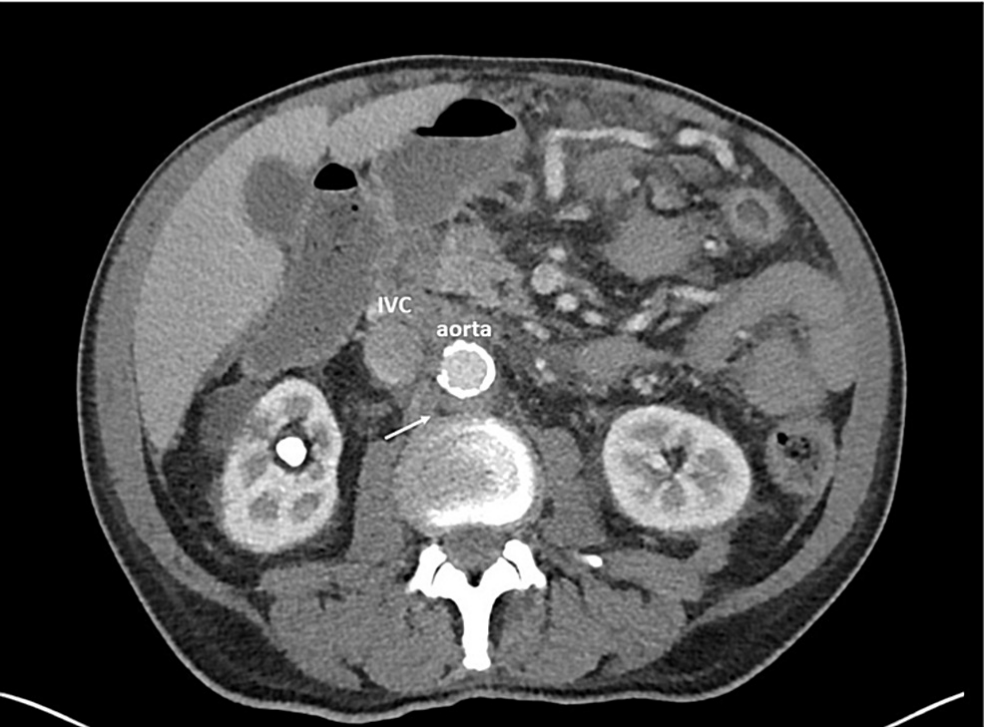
CT scan of the abdomen showing bulky paraaortic lymphadenopathy (white arrow) CT: computed tomography
